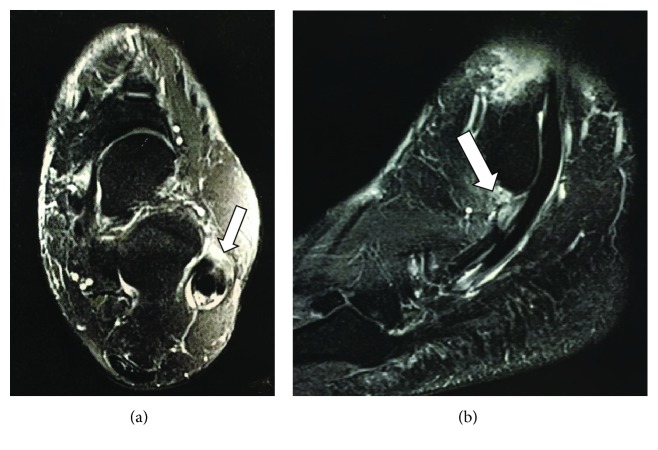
MRI showing PB tendon tears greater than 50% in the (a) axial and (b) sagittal views (white arrows).
